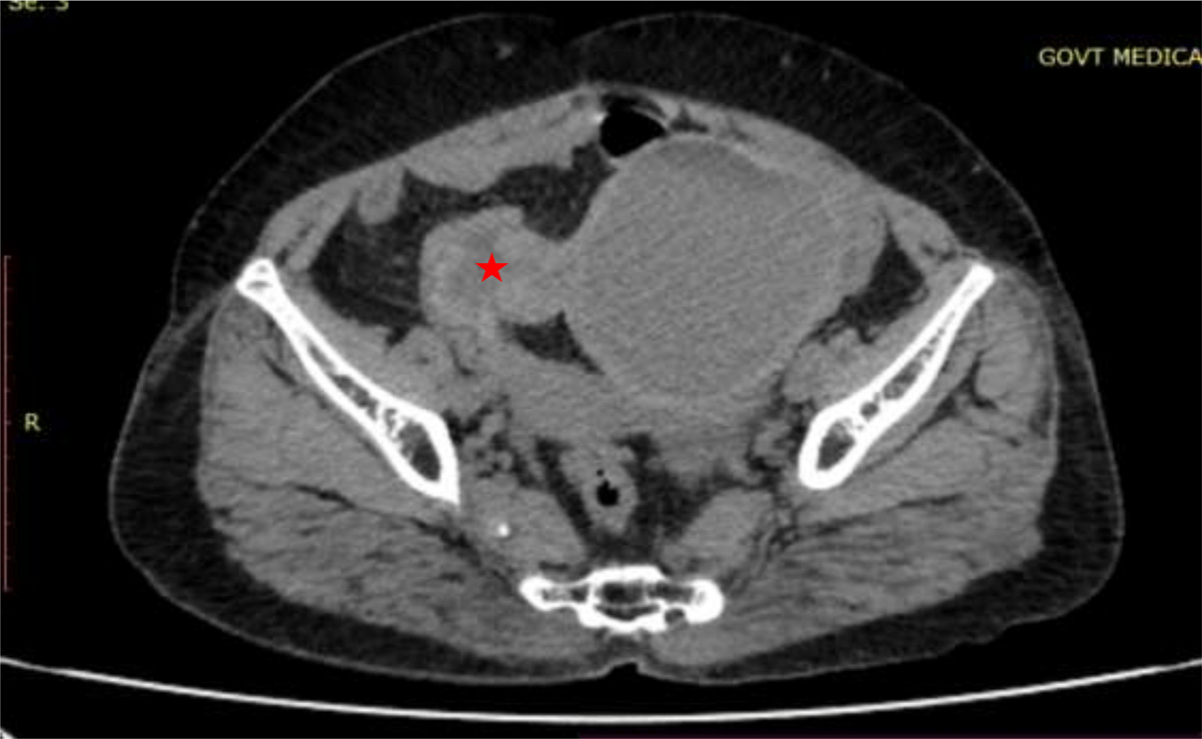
Axial CT image shows the pedicle of the lesion appearing thickened, edematous and twisted (red star).
